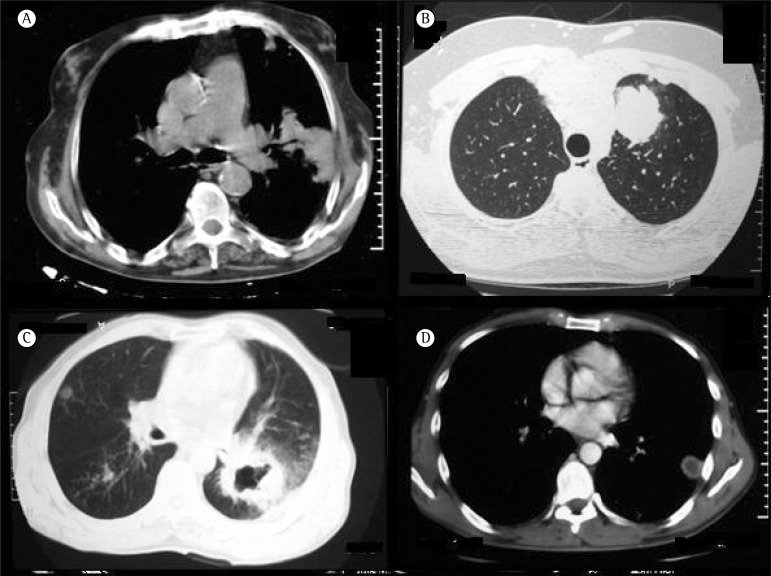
Helical CT scans showing an irregular lung mass (in A; male patient, 77
